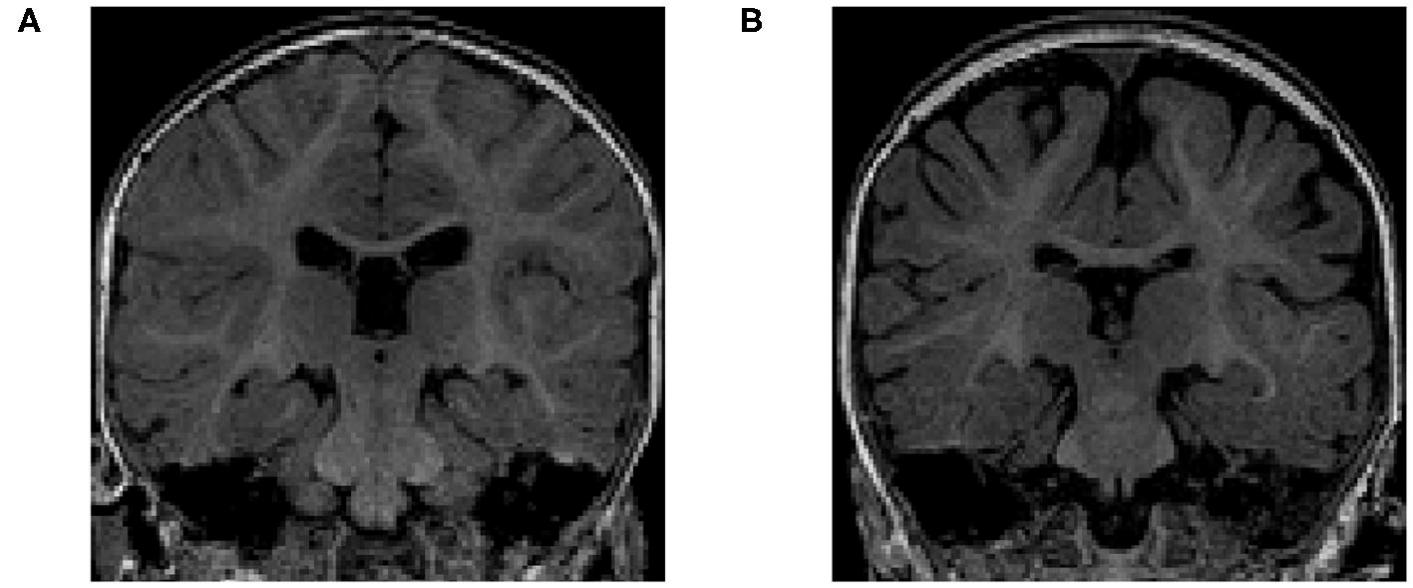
(A) T1-weighted MRI of a typically-developing infant with a normal MRI at 6 months of age. (B) T1-weighted MRI of an infant with enlarged EA-CSF volume at 6 months of age, who was diagnosed with ASD at 2 years of age. The dark regions between the brain folds and skull indicate increased volume of EA-CSF.
